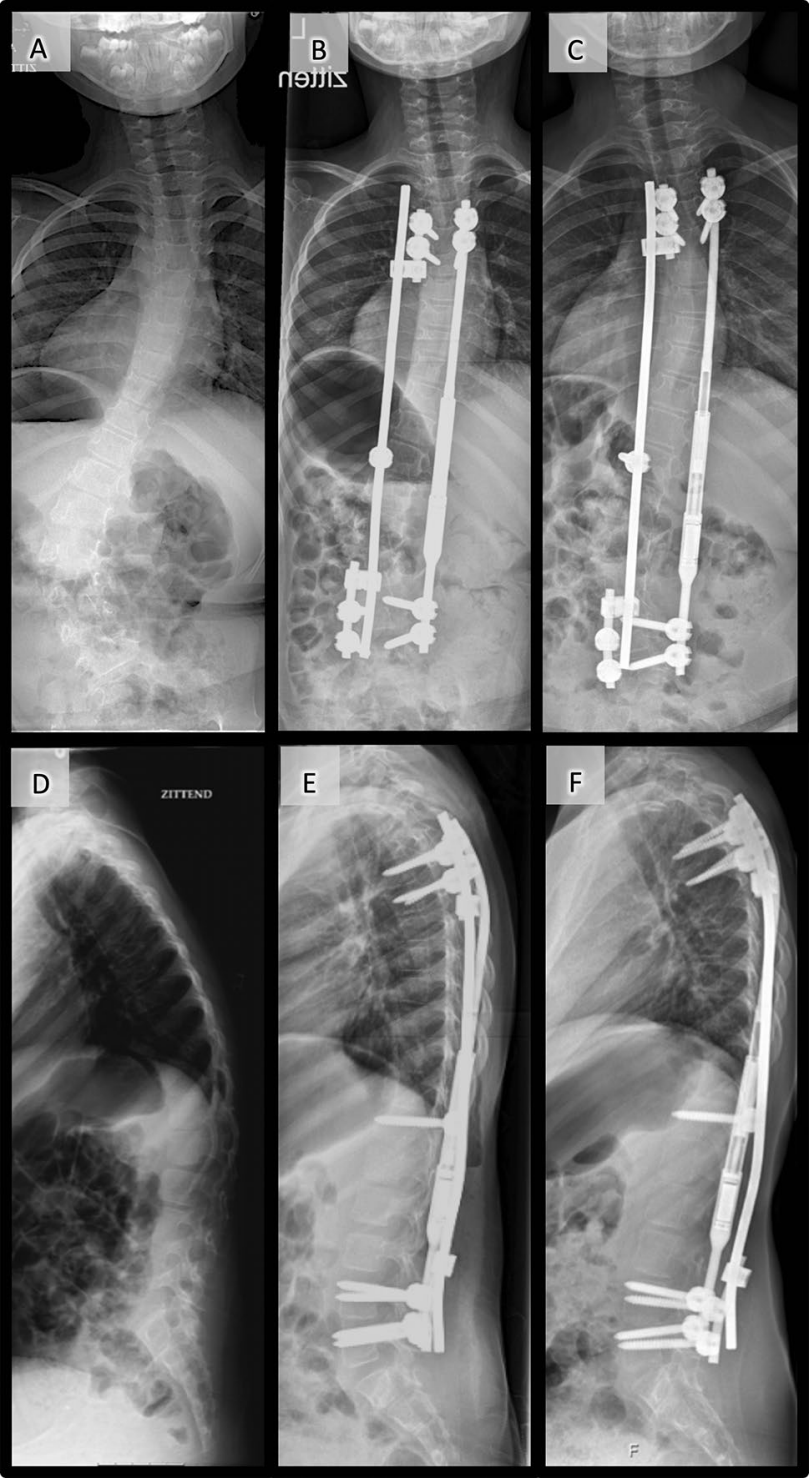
Combined single magnetic rod and parallel block sliding rod system in a 7-year-old girl with spinal muscular atrophy type 2: a frontal radiographs made preoperatively, b postoperative, and c at the time of final follow-up, and d sagittal radiographs made preoperatively, e postoperative, and f at the time of last follow-up
